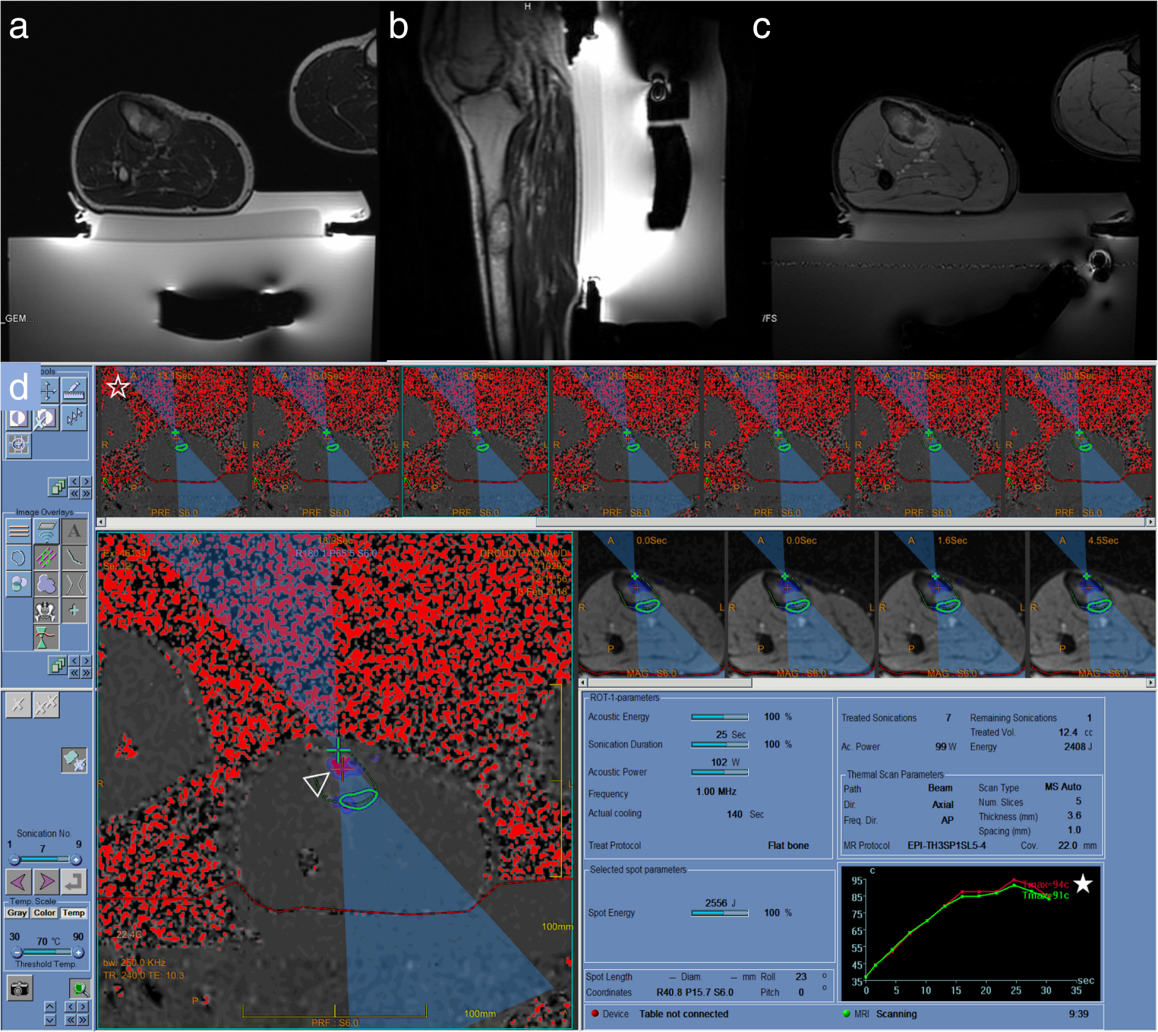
Patient 13. a, b Axial and Sagittal T2-weighted MR image showing a bone metastasis of the tibial diaphysis in front of the transducer. c Axial T1-weighted fat-suppressed with contrast MR image showing thermal destruction and necrosis inside the lesion. d Screen capture during sonication showing the thermal dose deposit (hollow arrowhead) inside the diaphysis. Thermal monitoring is performed using a map (empty star) and a graph (star). Temperature monitoring (star) shows a mean temperature of 91 °C at the end of this sonication
